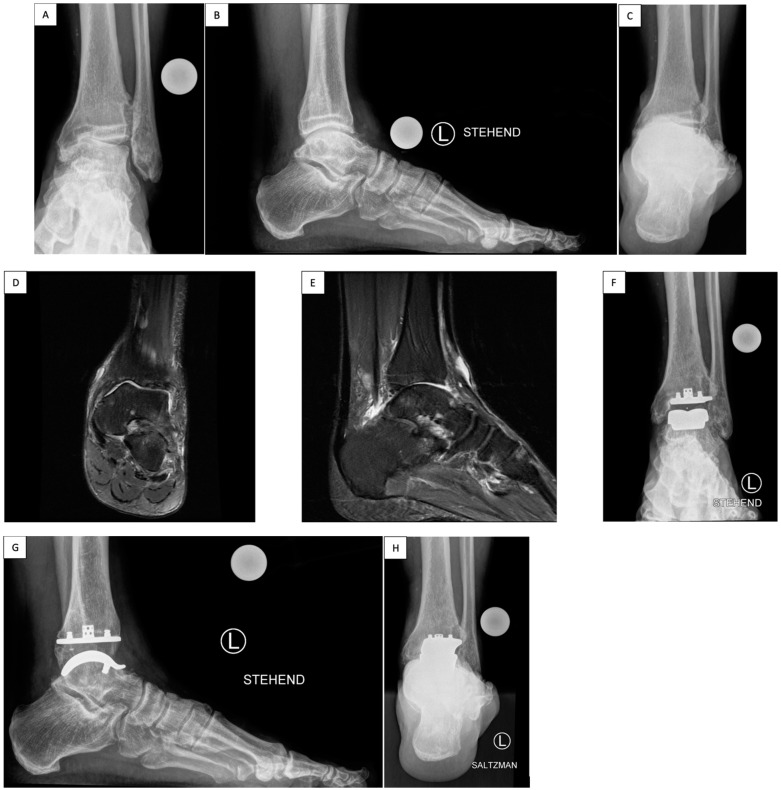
Sports-active patient with VANTAGE Total Ankle Arthroplasty mobile-bearing system. A 59-year-old patient suffered from post-traumatic ligamentous end-stage varus ankle osteoarthritis (OA) with chronic lateral ligament ankle instability and peroneus brevis tendon lesion (A–C, pre-op). The preoperative MR images show the medial predominantly ankle OA (D,E, pre-op). The surgery comprised TAA implantation, deltoid release, lateral ankle ligament reconstruction, and peroneus longus (PL)-to-brevis (PB) tendon transfer. After TAA surgery at recent 3-year follow-up he reached an AOFAS Ankle/Hindfoot Score of 100, VAS Pain Score of 0, Dorsiflexion/Plantarflexion 25-0-40, and Inversion/Eversion 30-0-10 (F–H); could return to a sports level of >5 h/week including hiking over 10 km and skiing; and obtained a Subjective Patients’ Satisfaction Score of 10 points (very satisfied). He describes a sense of the “forgotten joint” level. The radiological examination shows at 3-year follow-up a well-osteointegrated TAA with no signs of lucency or loosening.
